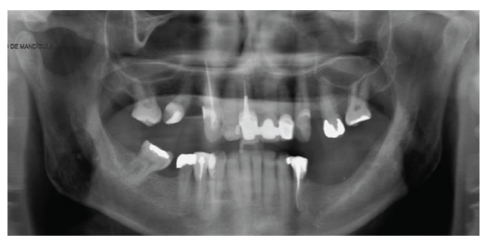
Panoramic radiograph did not reveal bone defects in the mandible.
